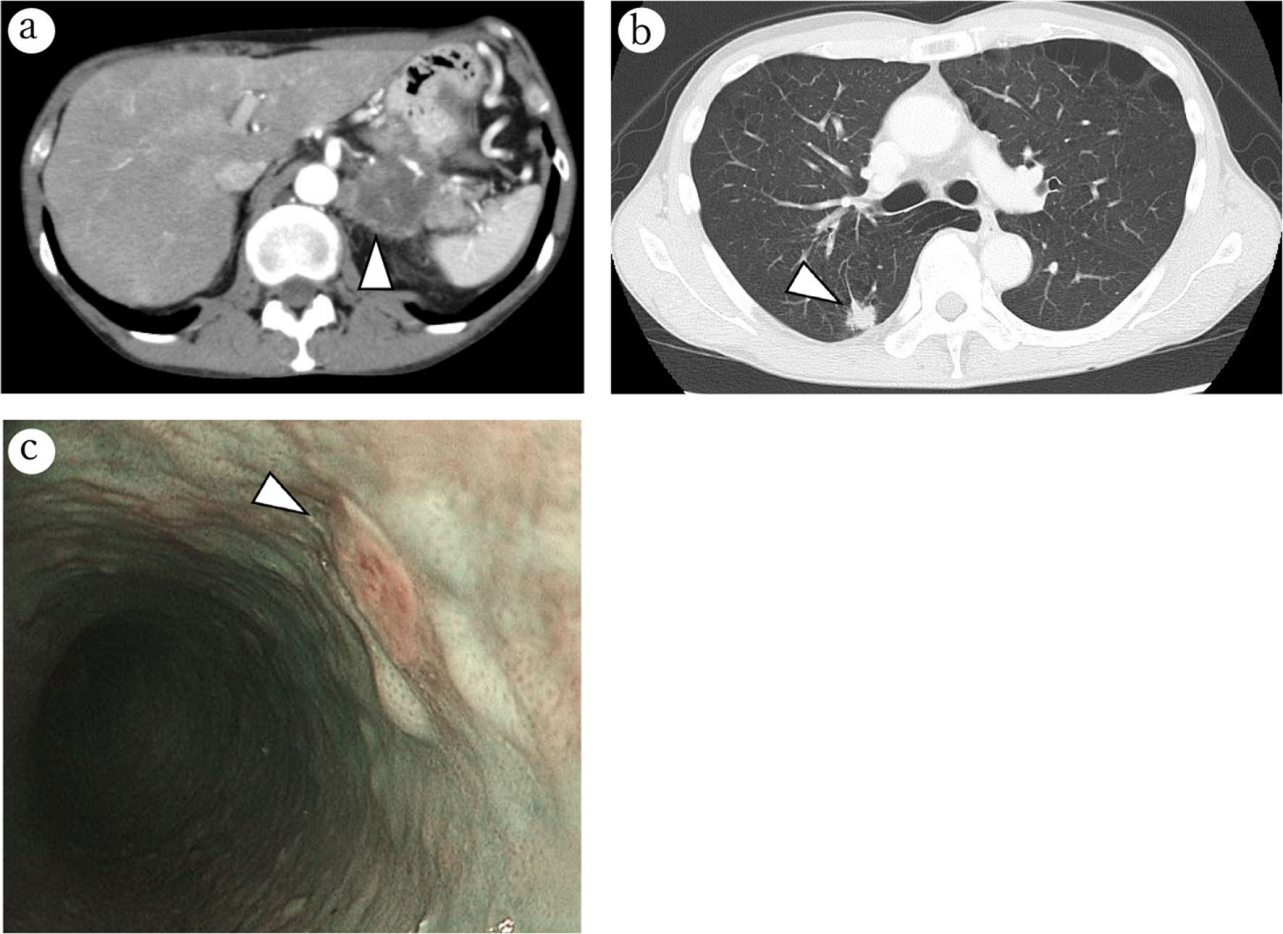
CT and upper gastrointestinal endoscopy findings at presentation. Preoperative CT showed an irregular-shaped mass 55 mm in size at the tail of the pancreas, which was suspected to have caused aortic invasion (arrowhead; a). CT showed a tumor of the right S2/6, 17 mm in size (arrowhead; b). Upper gastrointestinal endoscopy showed flat early esophageal cancer (0–IIb) located ~ 30 cm from the incisor teeth (arrowhead; c)
